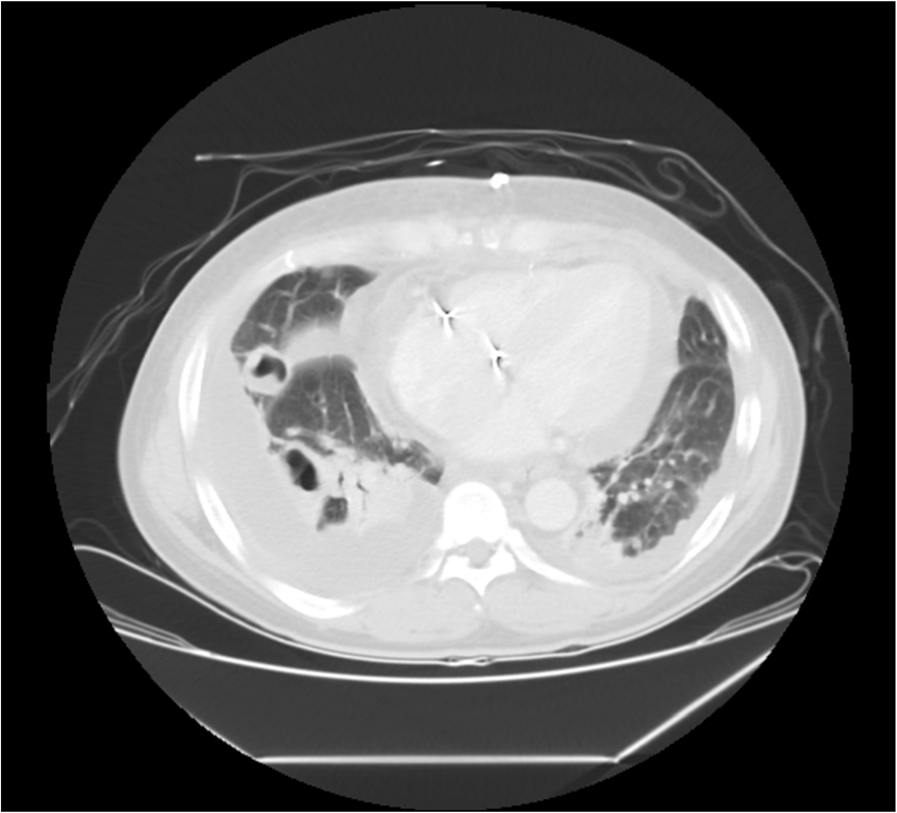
Chest CT reveals multiple cavitary nodules in the bilateral lungs
